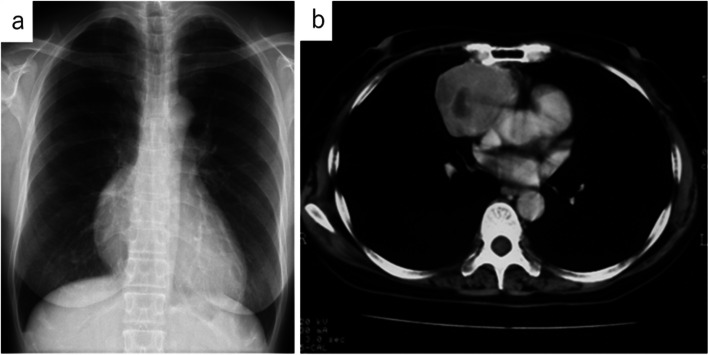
a Chest X-ray before the initial surgery. A solid mass with a clearly defined border can be observed in the right lower hilum, showing a positive silhouette sign at the border of the right atrium. b Enhanced chest computed tomography scan. An 80-mm solid mass with a clearly defined rim in the anterior mediastinum is observed
